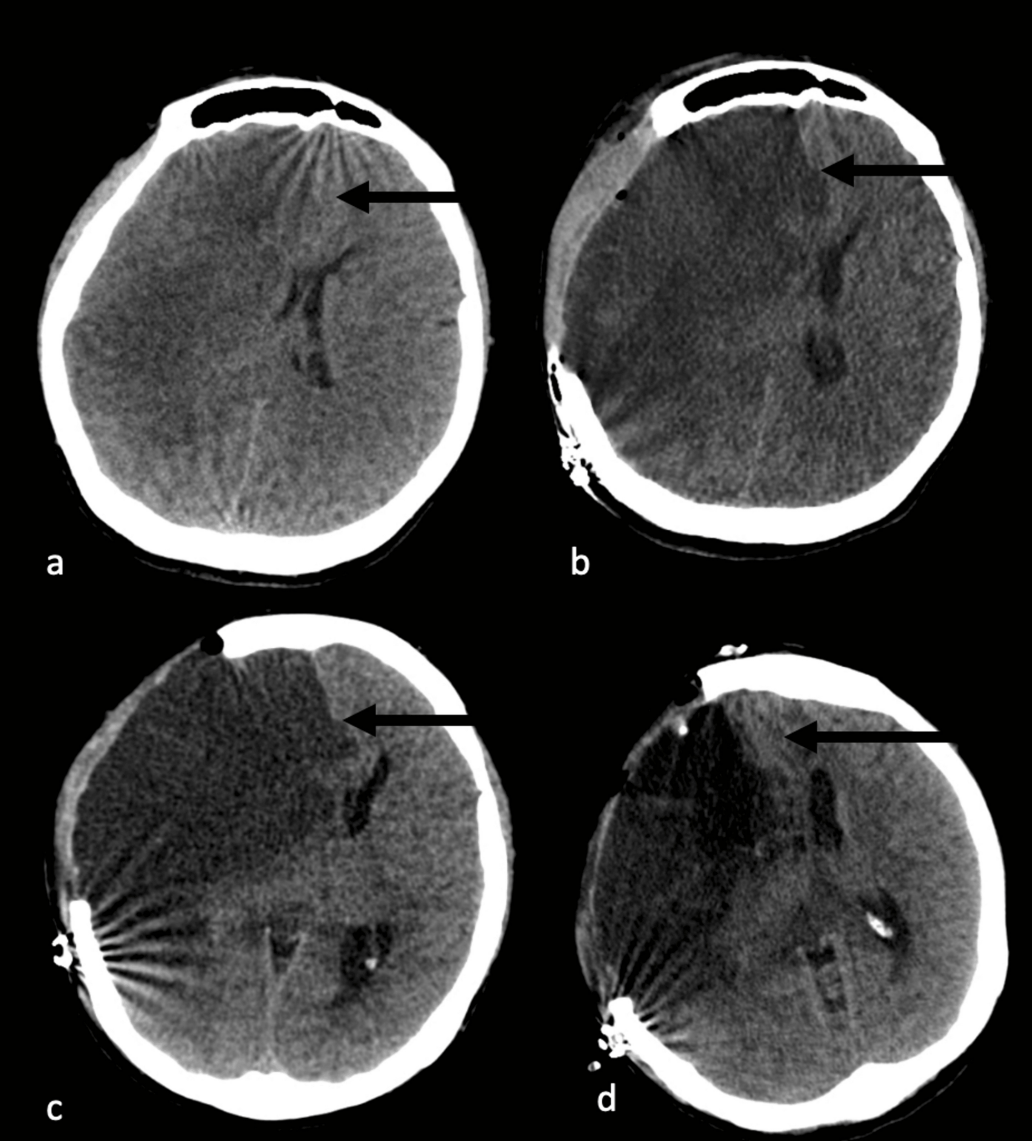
Serial brain computed tomographies of the patient (a) before decompressive craniotomy, (b) 48 hours after craniotomy, (c) 72 hours after craniotomy, and (d) 48 hours after partial frontal lobectomy (black arrows)
